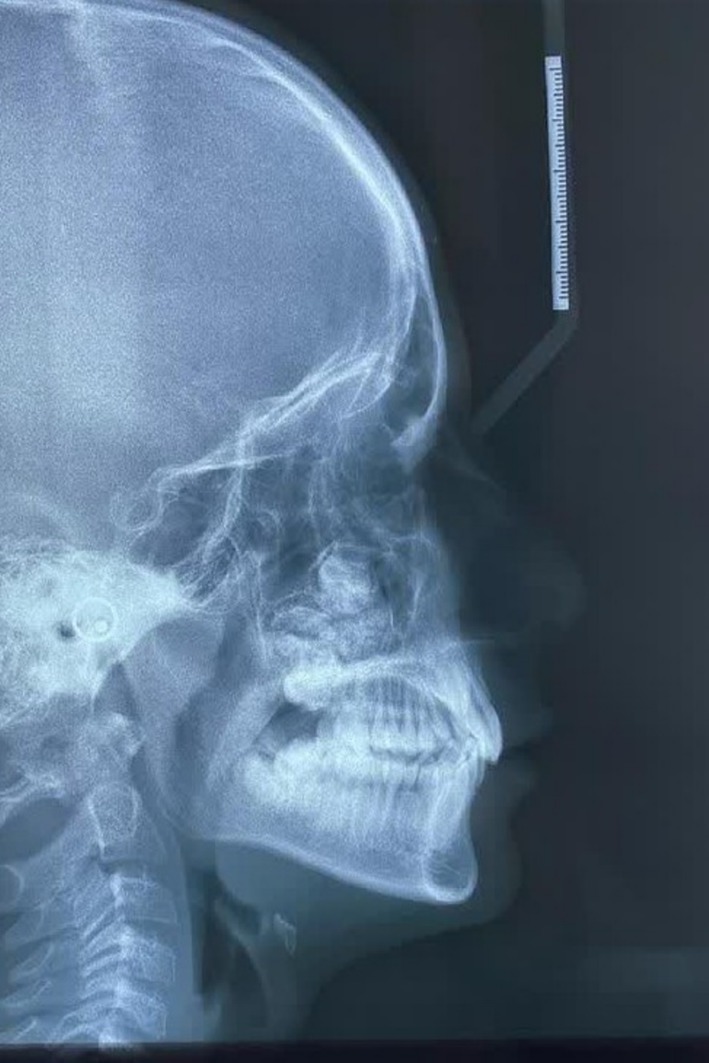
Lateral cephalometric radiograph showing a well‐defined mixed radiolucent–radiopaque lesion in the posterior maxilla.
